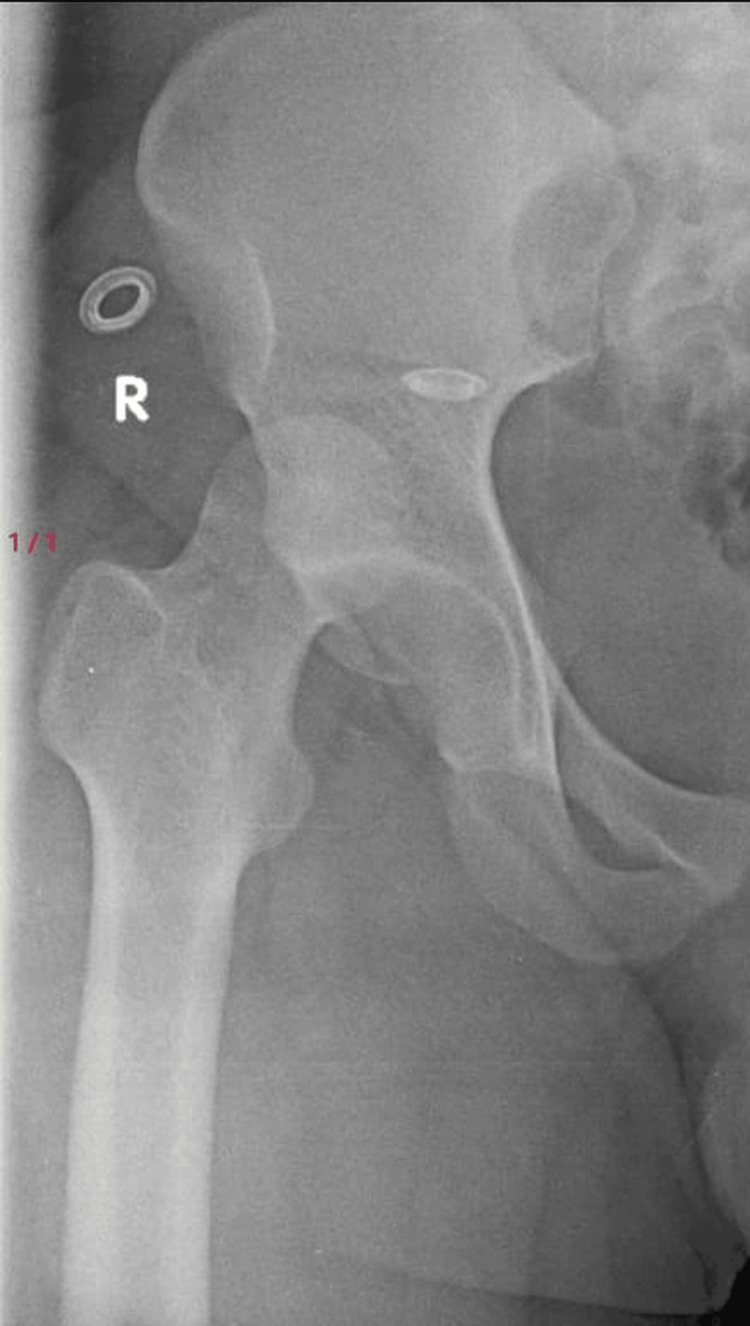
Pre-reduction anteroposterior radiographic view of the right hip on initial presentation. The radiograph demonstrates posterior dislocation, with the femoral head displaced superolaterally, lying outside the acetabular contour (orientation marker R denotes right side). Shenton’s line is disrupted on the right. There is no femoral neck or acetabular fracture appreciable on this initial screening view.
